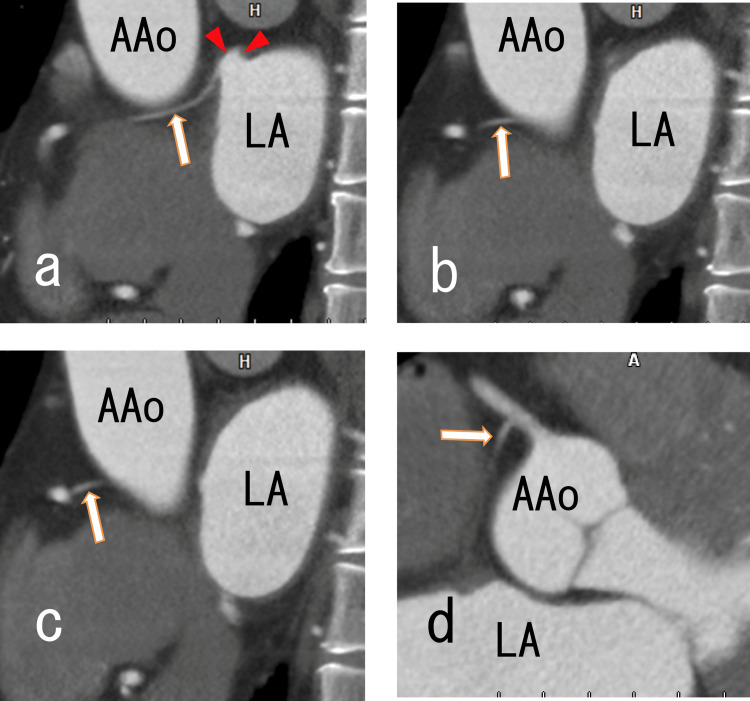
Sagittal and axial images from an 80-MDCT scan revealing a branch of the RCA (#1) that was connected to the LAD Sagittal and axial images from an 80-MDCT scan revealed a branch of the RCA (#1) that connected to the LAD. a. Sagittal images revealing a thin branch (arrow) connected near the top area of the LAD (arrowheads). b. Sagittal images revealing the thin branch (arrow). c. Sagittal images revealing that the thin branch was connected to the RCA (#1) (arrow). d. Axial images revealing the thin branch of the RCA (#1) (arrow). 80-MDCT, 80-slice multidetector computed tomography; RCA, right coronary artery; LAD, left atrial diverticulum; AAo, ascending aorta; LA, left atrium.
